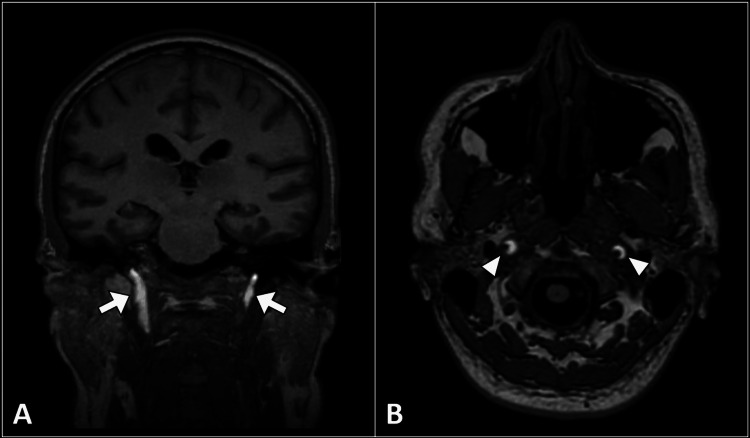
T1-weighted MR images in coronal (A) and axial (B) planes reveal bilateral acute hematomas in the internal carotid arteries (arrows). Notably, the crescent sign is depicted (arrowheads), indicative of vascular dissection. MR: magnetic resonance
